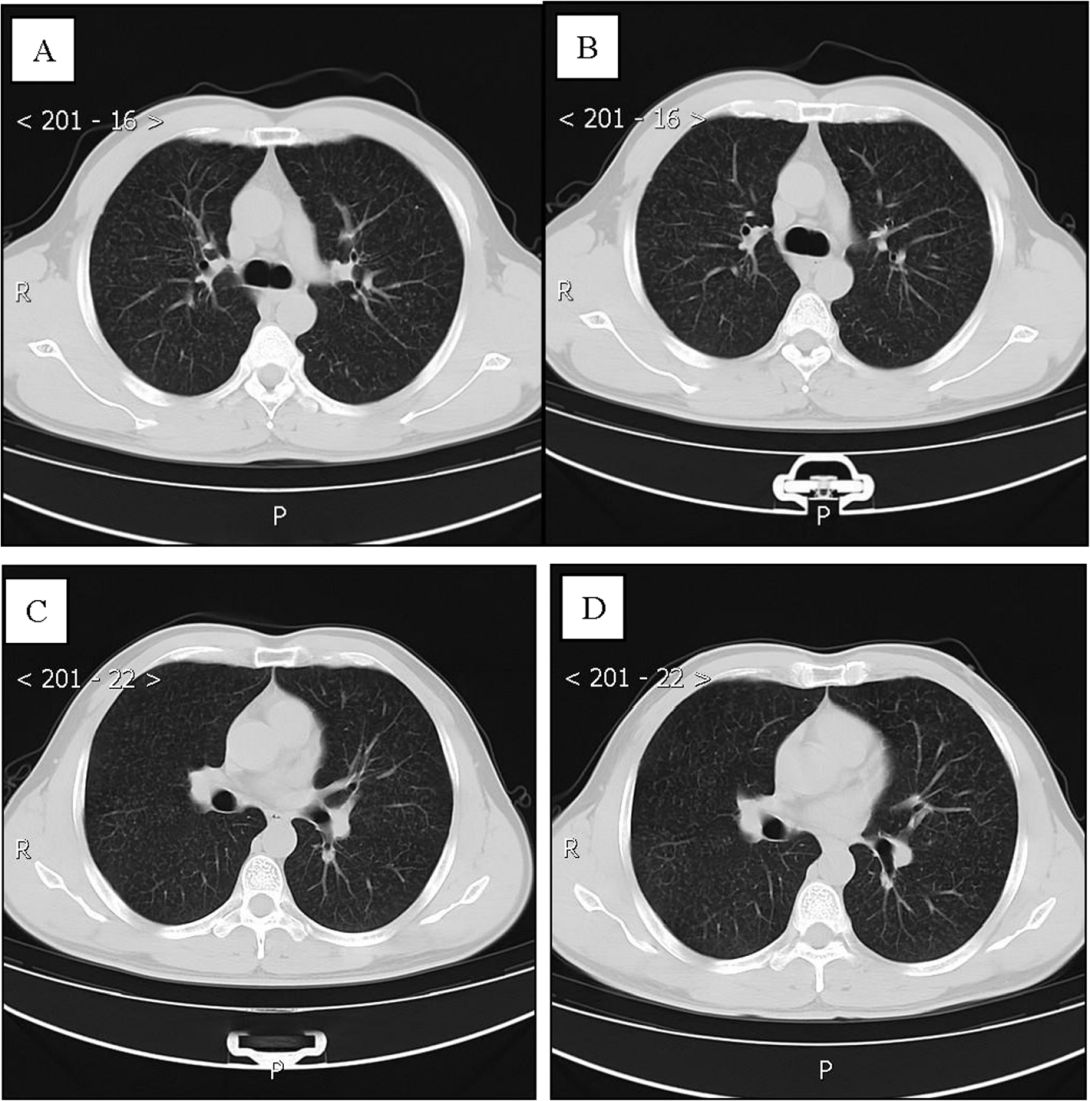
Chest CT images of A 43-year-old man with COVID-19 infection and pneumoconiosis. a, c Transverse chest CT images showed diffuse multiple nodules in both lungs on admission. b, d Transverse chest CT images showed diffuse multiple nodules in both lungs on discharge
